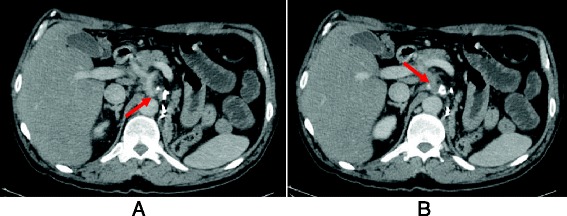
Assessment of response CT scan. CT scan performed at month 24 showing partial response to treatment as per RECIST 1.1 criteria: left coeliac lymph node measuring 1.0 cm in the short axis (a) and soft tissue mass next to the superior mesentery artery measuring 3.2 cm (b)
